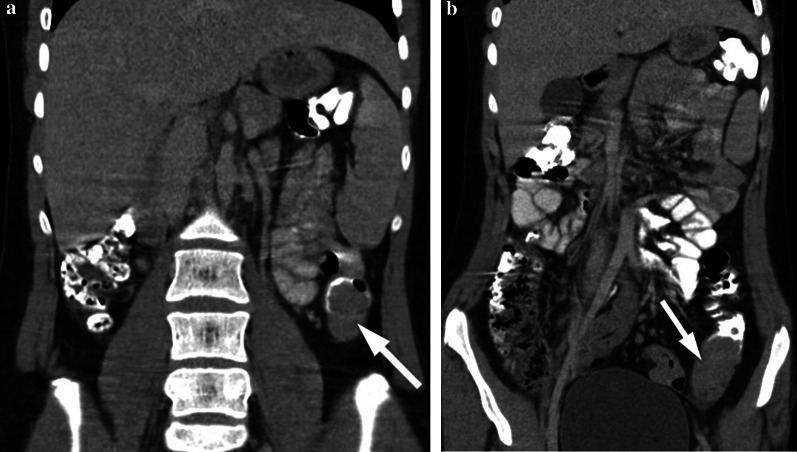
a, b A 17-year-old girl with rectal bleeding for the last 2 weeks. Hb level was 9.8 gr/dL (normal range 11.7–15.5 gr/dL). Colonoscopy (performed in an external center) showed many irregular mucosal polyps in the descending/sigmoid colon and rectum. Biopsy revealed villous adenocarcinoma. The patient underwent total colectomy. a, b Contrast-enhanced coronal reformatted CT images show hypodense polypoid tumoral lesions in the descending colon (arrows)
